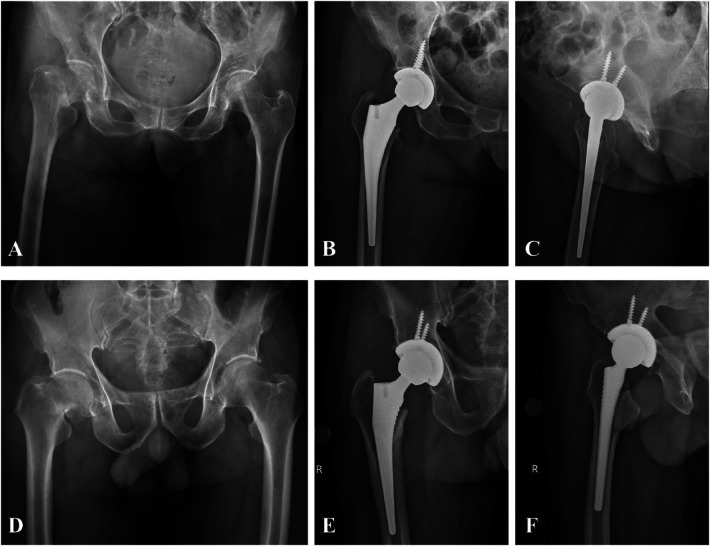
Perioperative DR results of typical cases. (A) Preoperative anteroposterior pelvis radiograph (Case 1). (B) Postoperative anteroposterior hip radiograph (Case 1). (C) Postoperative lateral hip radiograph (Case 1). (D) Preoperative anteroposterior pelvis radiograph (78M, right femoral neck fracture). (E) Postoperative anteroposterior hip radiograph (Case 2). (F) Postoperative lateral hip radiograph (Case 2).
